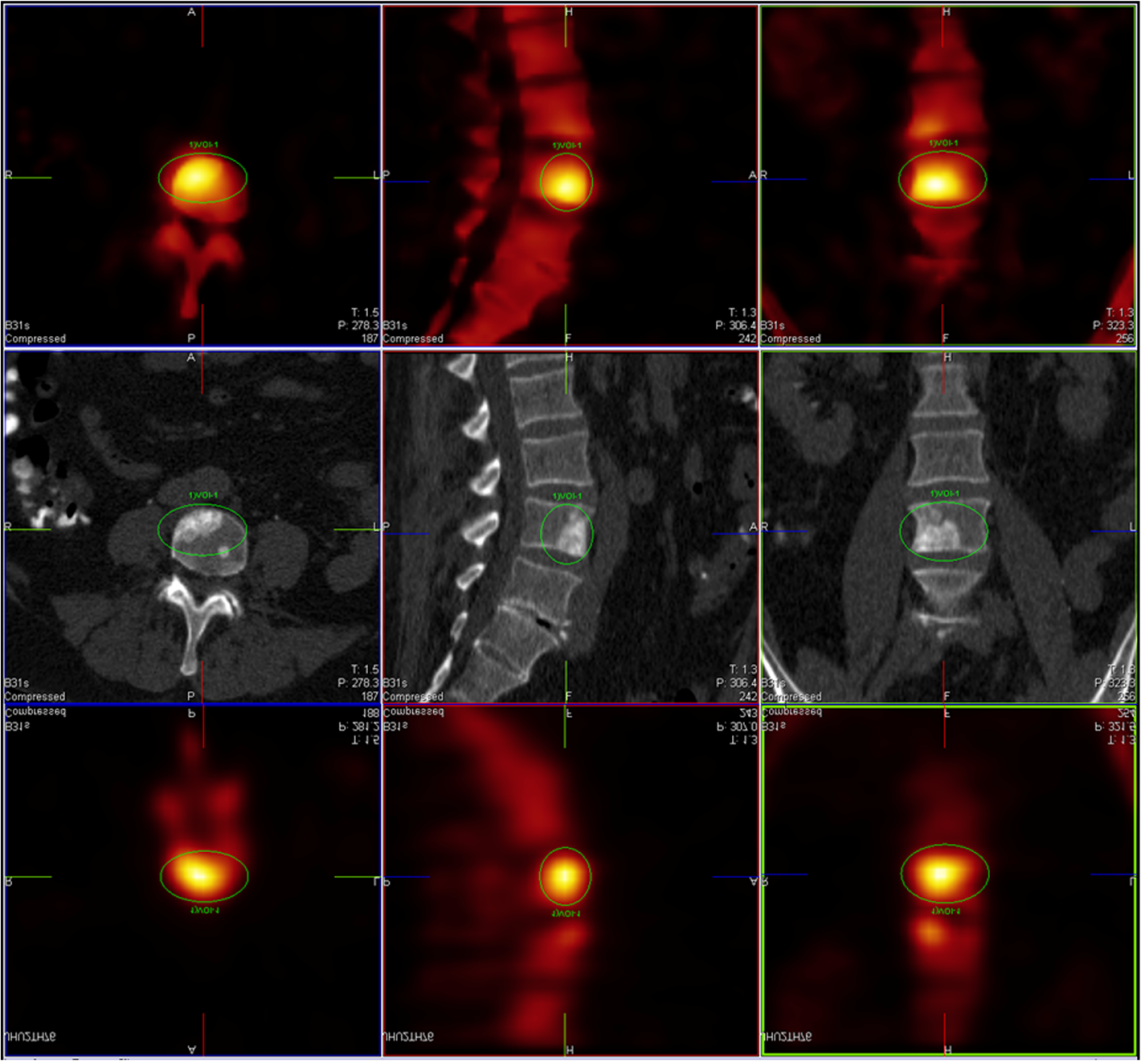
Reduction of false negative reads with xB. Here, a clearly visible lesion, read as benign by two readers in F3D, and as malign by all readers in xB, with confirmation by CT. [JHUTH76 L1]
